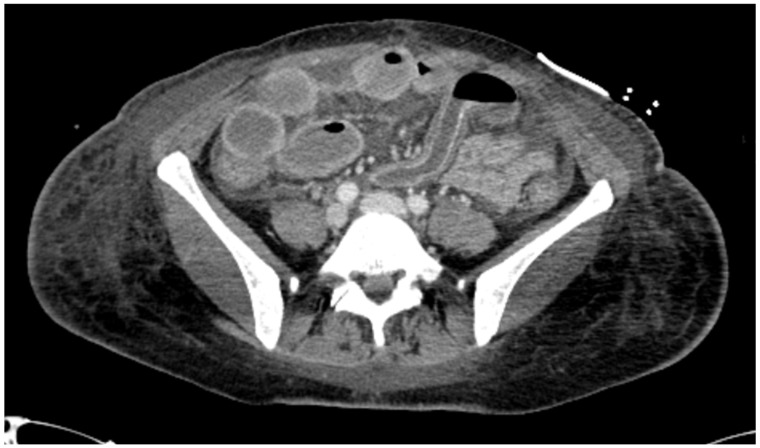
The CT scan showed diffuse bowel ischemia.
